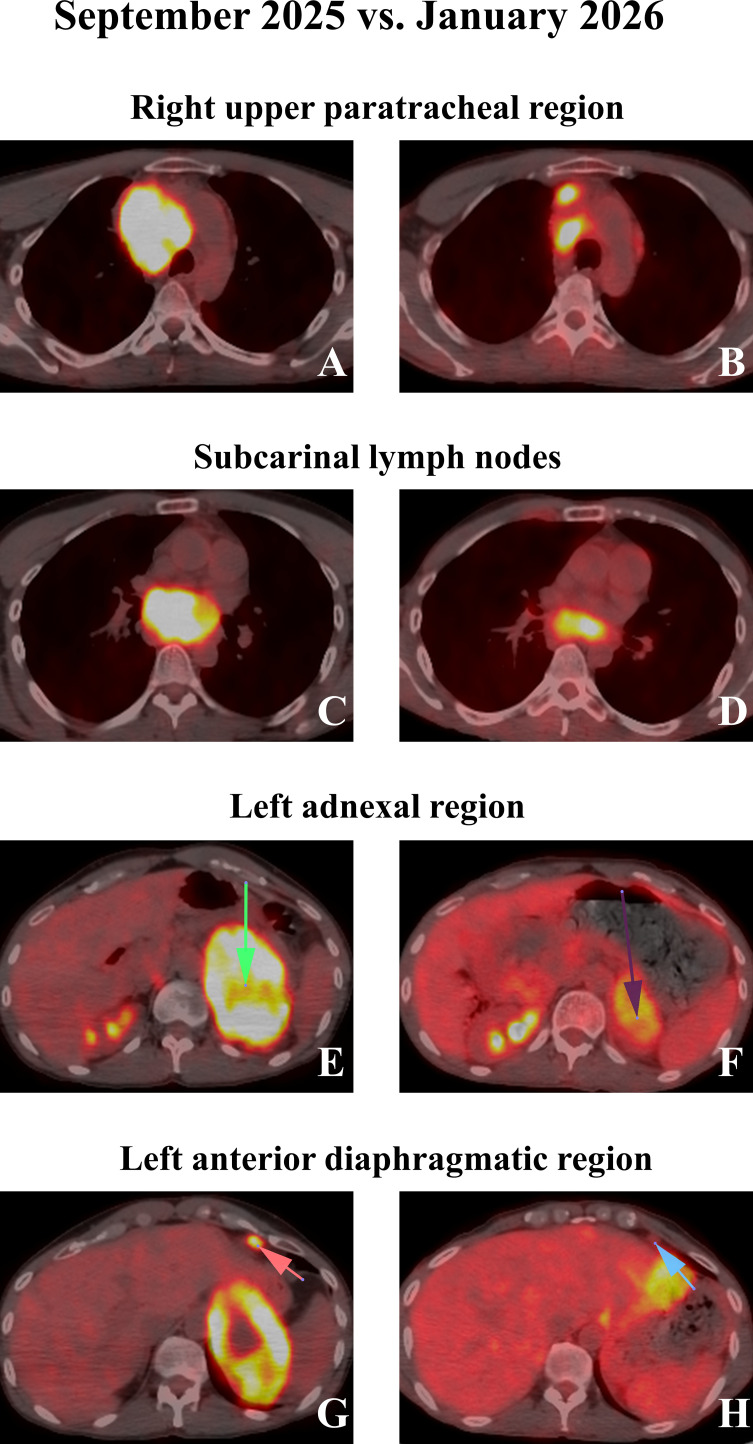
Longitudinal response evaluation. (A) Pre-treatment/baseline PET/CT imaging (September 2025) reveals a highly FDG-avid conglomerate lymphadenopathy in the right upper paratracheal region, encasing adjacent vascular structures and causing rightward tracheal deviation. (B) Post-treatment scan (January 2026) demonstrates marked regression of the primary mediastinal mass. (C–H) Comparative baseline and follow-up imaging highlighting significant anatomic and metabolic regression across multiple metastatic sites, including the subcarinal lymph nodes (C, D), the left adnexal region (E, F), and the left anterior diaphragmatic region (G, H).
